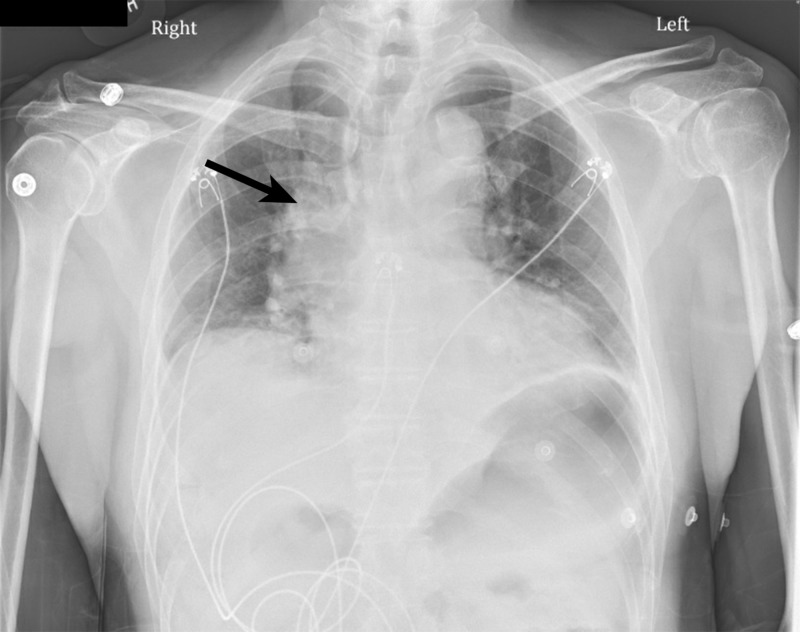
CXR with fullness of the right hilum CXR: chest X-ray
